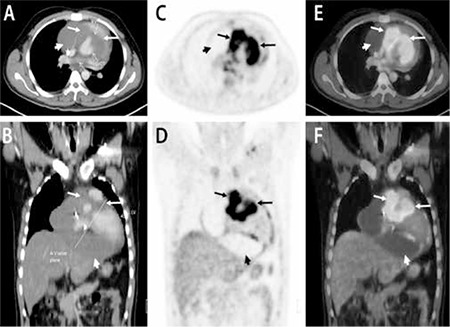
Contrast enhanced CT (Transaxial A, Coronal B) revealed a large ill defined non enhancing soft tissue density mass (bold arrows) in the interventricular septum (proximal two-third) extending into the right ventricle upto right ventricle outflow tract, the aortic root and the right A-V valve with a small extension along the left ventricular wall. 18F-FDG PET (Transaxial C, Coronal D) and contrast enhanced 18F-FDG PET-CT (Transaxial E, Coronal F) shows increased 18F-FDG uptake in the soft tissue mass seen on contrast enhanced CT (arrows, SUVmax-8.3). Also noted is loculated pericardial effusion without significant 18F-FDG uptake on 18F-FDG PET (arrowheads).RV-right ventricle; LV-left ventricle; RVOT-RV outflow tract; LVOT-LV outflow tract; A-V-atrio-ventricular.
